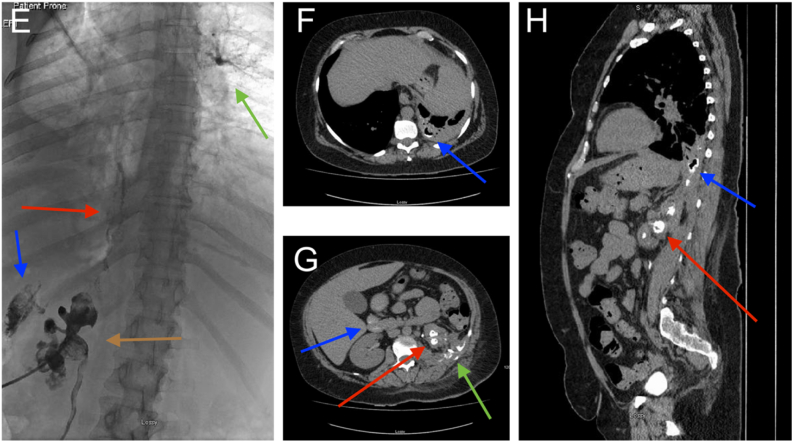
E: Fluoroscopic image from left nephrostogram (patient prone) demonstrating contrast filling left collecting system, with filling defects likely representing blood clots and debris (brown arrow), extrarenal contrast secondary to calyceal rupture (blue arrow), extrarenal contrast extending from upper pole to left hemidiaphragm (red arrow), contrast within the right bronchial tree, consistent with nephron-bronchial fistula (green arrow). The patient was noted to cough at time of nephrostogram F: Axial image from non-contrast enhanced CT immediately following nephrostogram demonstrating contrast pooling in cavitation within the atelectatic left lower lobe (blue arrow). G: Axial image from same non-contrast enhanced CT demonstrating contrast in the left renal calyces (red arrow), pararenal contrast secondary to ruptured calyces (green arrow), intraluminal contrast in the proximal duodenum secondary to aspiration (blue arrow). H: Axial image from same non-contrast enhanced CT demonstrating contrast in the left renal calyces (red arrow), contrast extending through defect in the left hemidiaphragm to left lower lobe cavitation and peripheral segmental bronchi (blue arrow). (For interpretation of the references to colour in this figure legend, the reader is referred to the web version of this article.)
